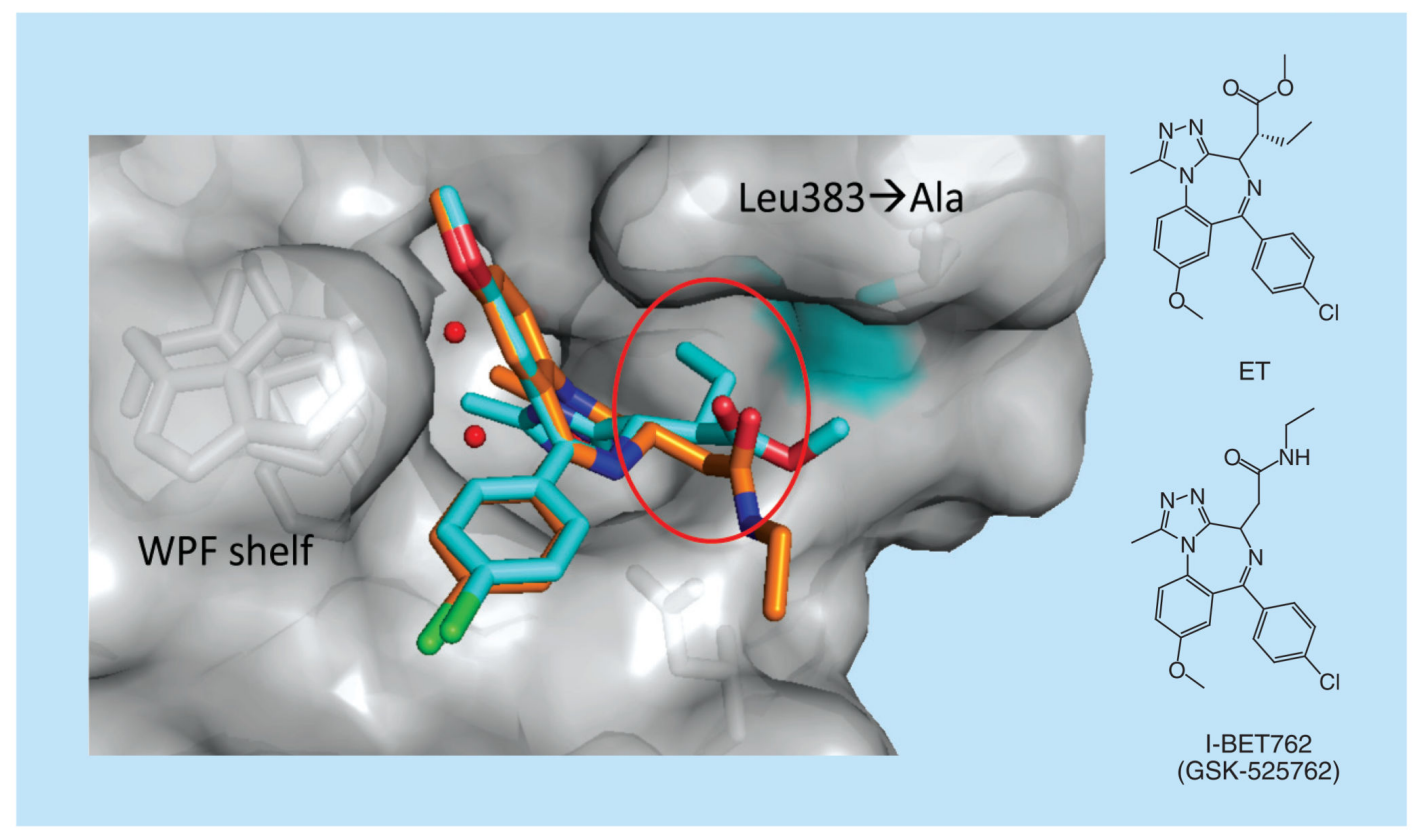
Bump-and-hole approach. Superposition of the x-ray structure of the ET (in cyan) bound to the BRD2-BD2 _L383A_ (in gray, PDB 4QEW) and parent ligand I-BET762 (in orange) bound to the BRD2-BD2 (not shown, PDB 5DFC).
